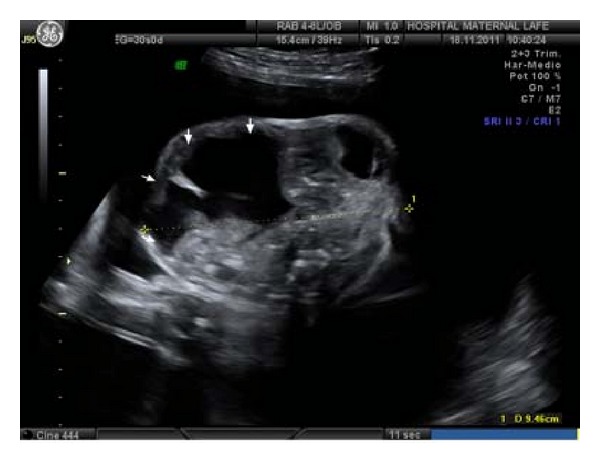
New ultrasound control in week 30, which maintains the increased tumor size (arrows) and heterogeneous component.
